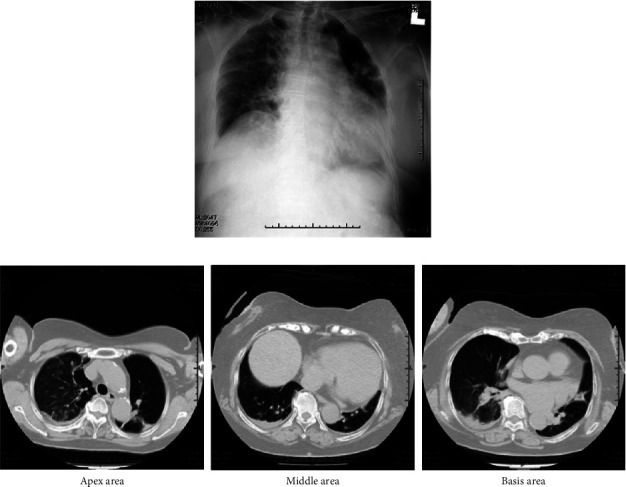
(a) Chest radiograph at the end of the surgery and (b) Chest computed tomography scan at the end of the surgery.
